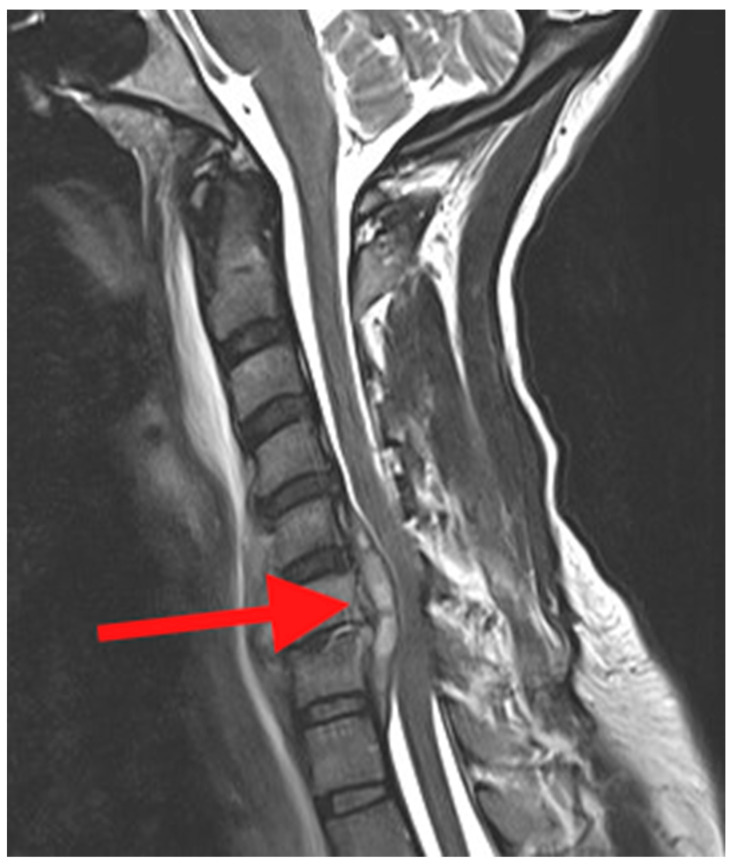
Sagittal T2 weighted MRI of the cervical spine. The red arrow points to an epidural abscess behind the C5, 6, and 7 vertebral bodies and compression of the ventral spinal cord.
